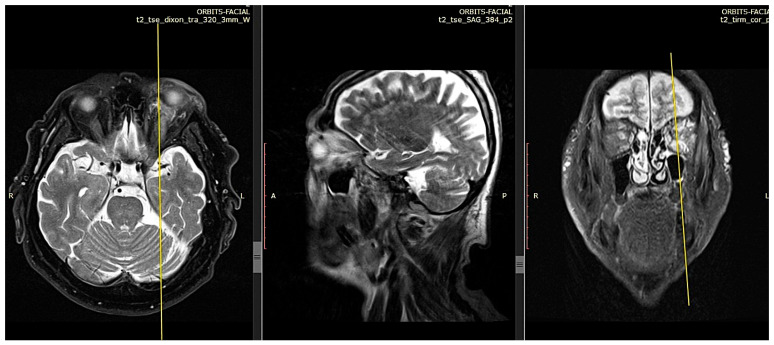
MRI, T2-weighted images from axial, sagittal, and coronal perspectives 8 months after initial treatment. Generalized thickening and deterioration of edema of all the extraocular muscles, mostly evident in the lower rectus muscle.
